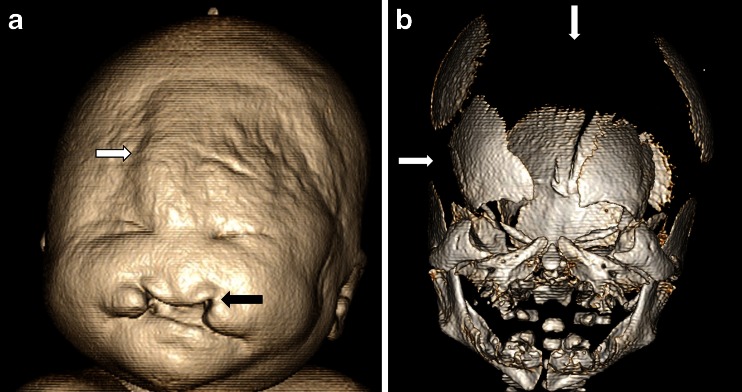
Postmortem CT of a male foetus who died at 22 weeks after a terminated pregnancy following antenatal sonographic diagnosis of holoprosencephaly and a midline facial defect. a Surface-rendered postmortem CT shows a cleft lip (*black arrow*), the presence of an interorbital proboscis (*white arrow*) and two eyes. b Three-dimensional reconstruction of the skull shows a midline defect with absence of bony medial boundaries of the orbits. Postmortem moulding has caused diastasis of the parietal and occipital bones (*white arrows*). Based on the imaging findings, a diagnosis of ethmocephaly was made; parental consent for autopsy was refused
